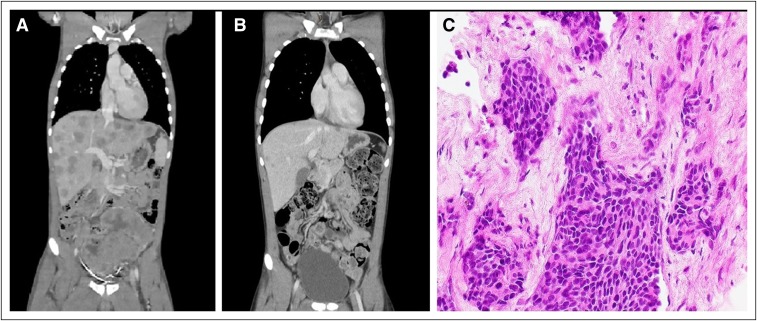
Computed tomography (CT) scan showing a large soft-tissue mass in the pelvis and multiple, hypodense lesions in the liver. (A) CT scan showing a large tumor in abdomen with hepatic metastasis before chemotherapy. (B) Markedly reduced tumor burden after three cycles of chemotherapy. (C) Characteristic desmoplastic small round cells with scanty fibrous stroma.
